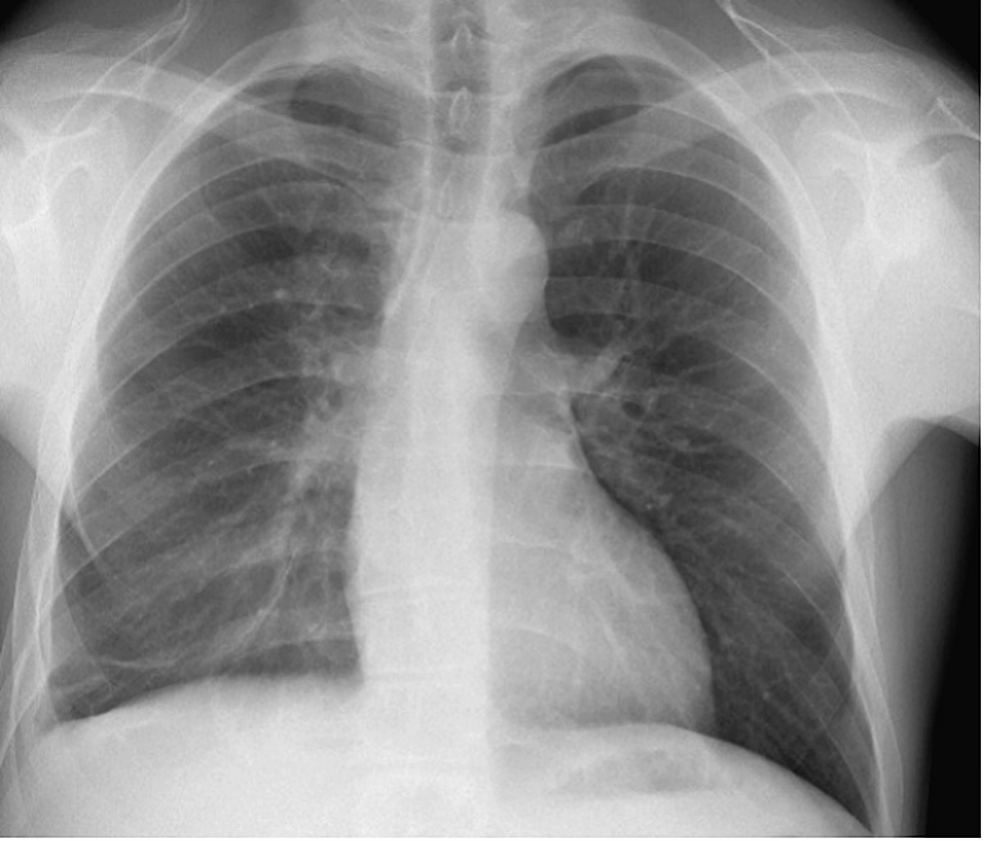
Chest X-ray taken three weeks after the previous film demonstrating resolution of infiltrates previously present on the left lung and new mild infiltrates in the right middle lobe.
